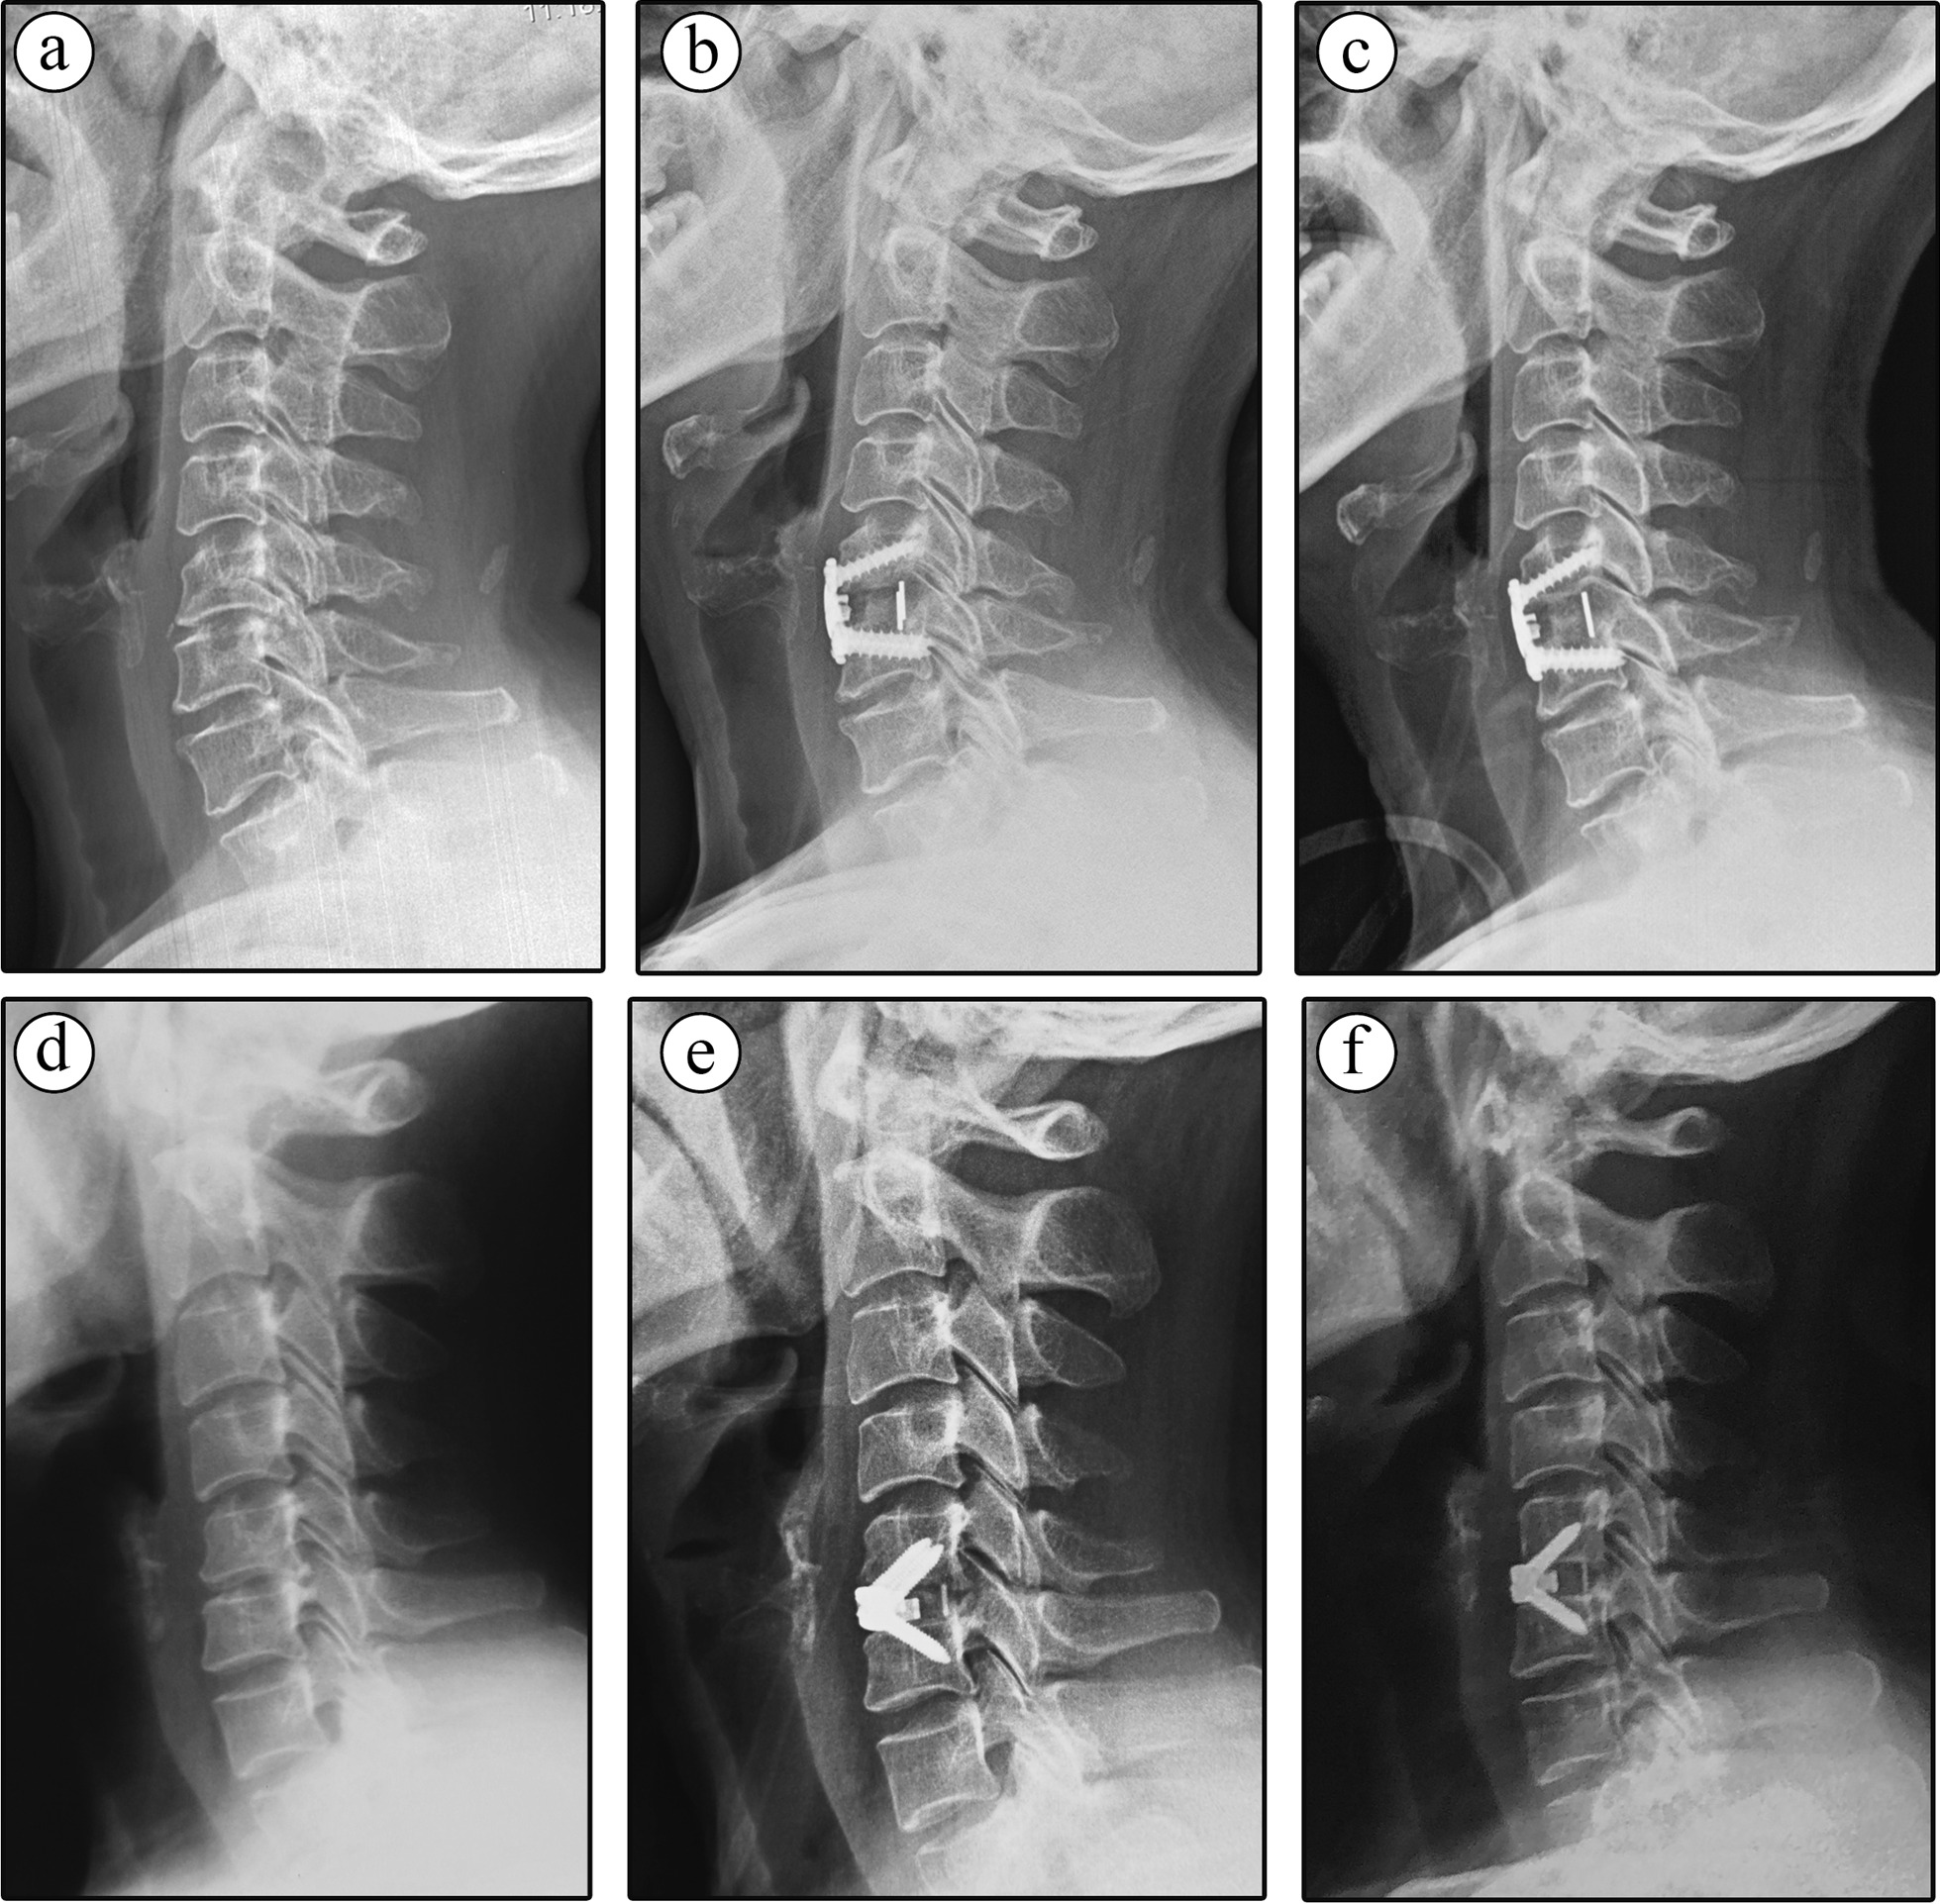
Representative cervical X-ray images of Carmen and Zero-P implant treated patient. a–c Single-level male patient treated with Carmen device, the segmental Cobb angle of C5–C6 changed from 11-degree lordosis to 18-degree lordosis after the surgery, and reached 20-degree at one-year follow-up, as well as the C2–C7 Cobb angle. d, e Single-level male patient treated with Zero-P device, the segmental Cobb angle of C5–C6 changed from 7-degree lordosis to 9-degree lordosis after the surgery, and decreased to 4-degree at one-year follow-up, as well as the C2–C7 Cobb angle (15-degree preoperative, 20-degree postoperative and 15-degree at one-year follow-up)
